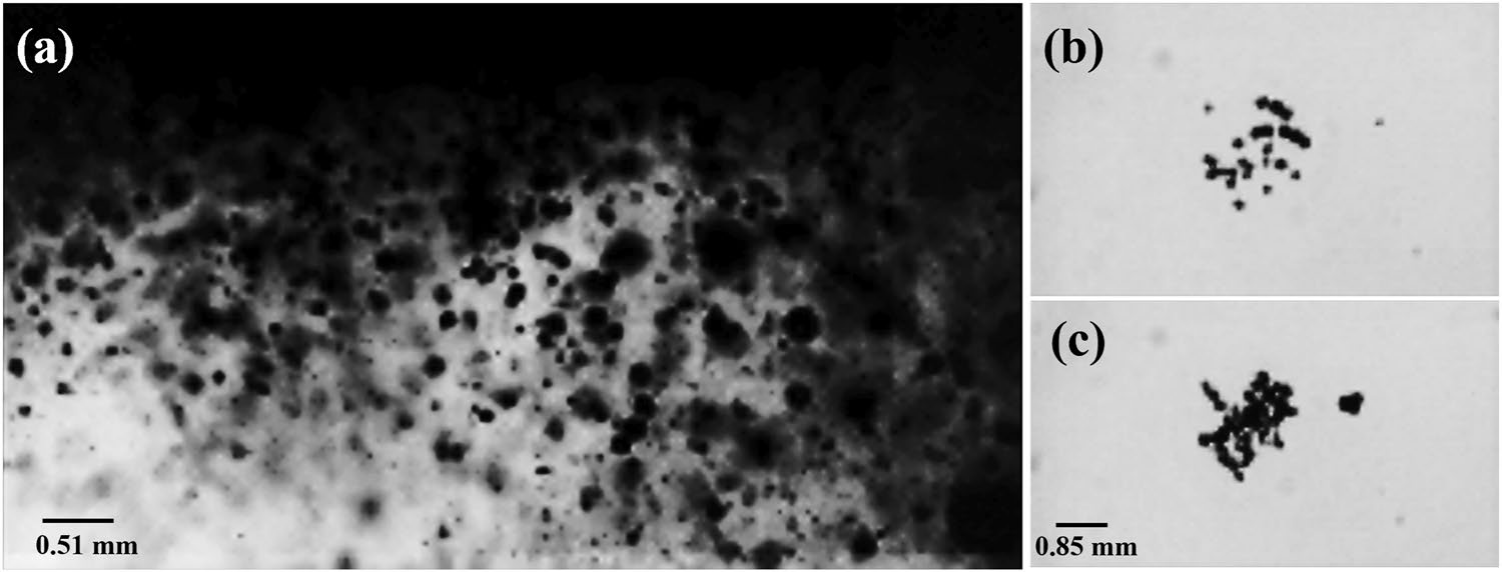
Cavitation bubbles formed by (a) a 20 kHz horn-type transducer, subjected to an ultrasound power of 70.43 W, captured near the tip of the probe using high-speed recording at 100k fps, (b) laser breakdown shockwaves (BSW), and (c) laser-induced bubble collapse shockwave (CSW), high-speed recordings at 60k fps.
